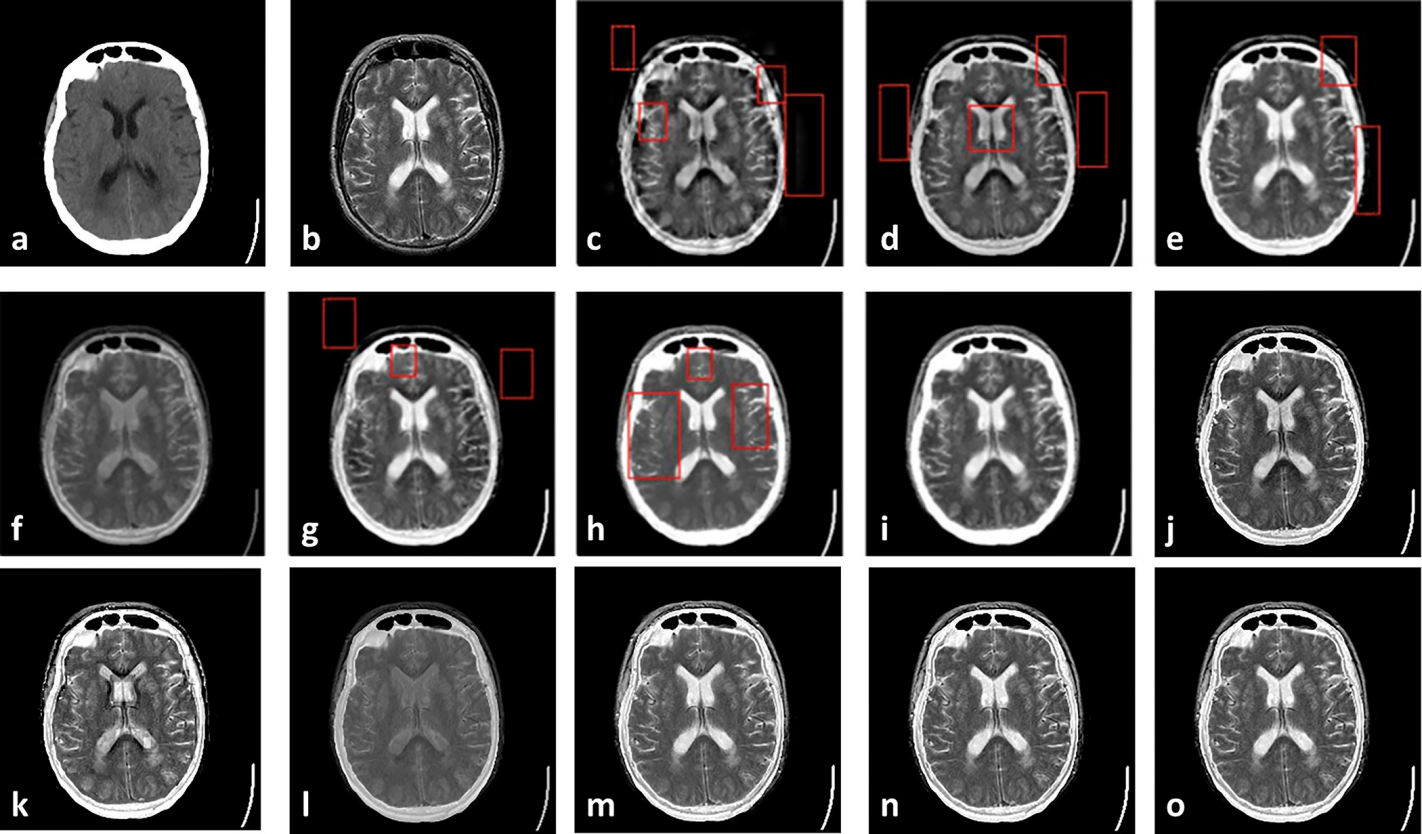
Experiment 1: Results of fusing CT and MR images of brain with acute stroke. (a) CT image; (b) MR-T2 image; (c) DWT; (d) NSCT; (e) NSCT-SR; (f) m-PCNN; (g) PCNN-NSCT; (h) SCM-F; (i) SCM-M; (j) Laplacian Pyramid; (k) Morphological Difference Pyramid; (l) PCA; (m) the proposed method with PCA, (n) max, and (o) weighted averaging model, respectively.
